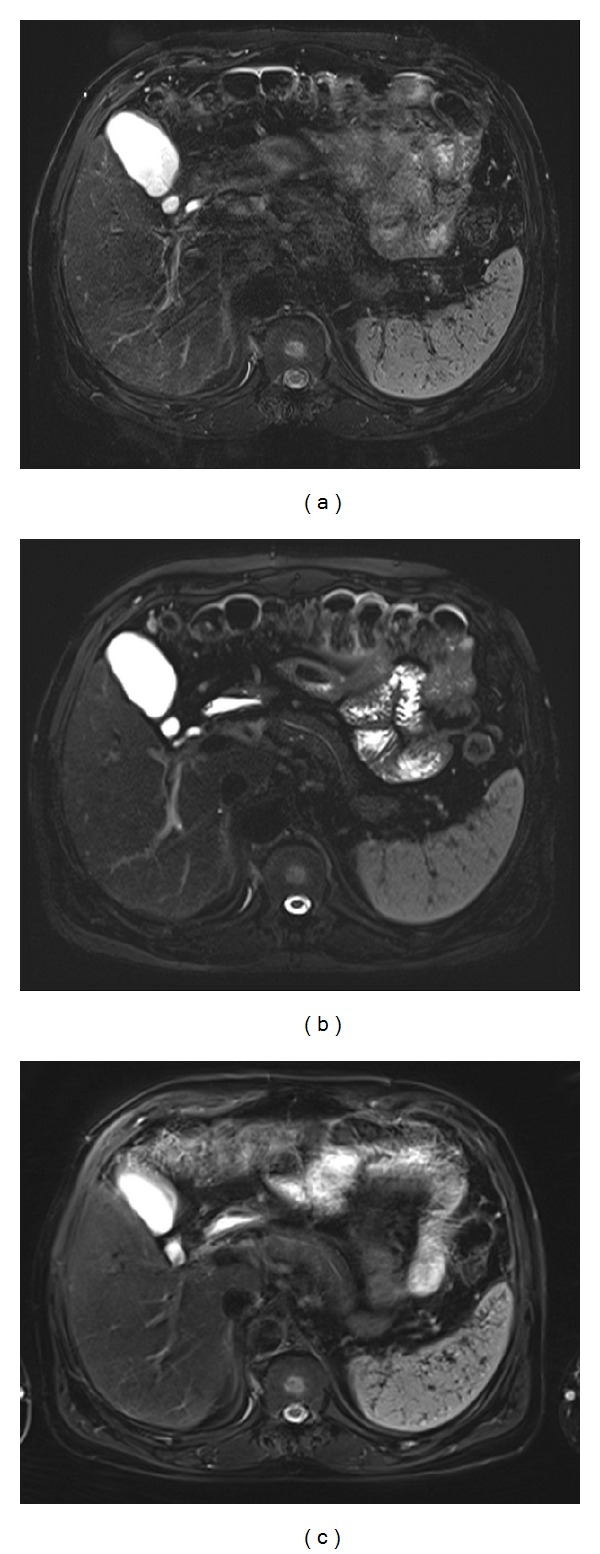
MR images obtained in a 53-year-old man with chronic liver disease. The conventional T2-weighted turbo spin-echo image (a) shows motion artifacts in the right posterior section of the liver. The artifact is improved on T2-weighted SS-ETSE (b) and BLADE (c) (proprietary name for periodically rotated overlapping parallel lines with enhanced reconstruction (PROPLLER) in MR systems from Siemens Medical Solutions) MR images. Much better sharpness of the liver edge and pancreas and clearer depiction of the main pancreatic duct are seen on T2-weighted SS-ETSE (b) and BLADE (c).
